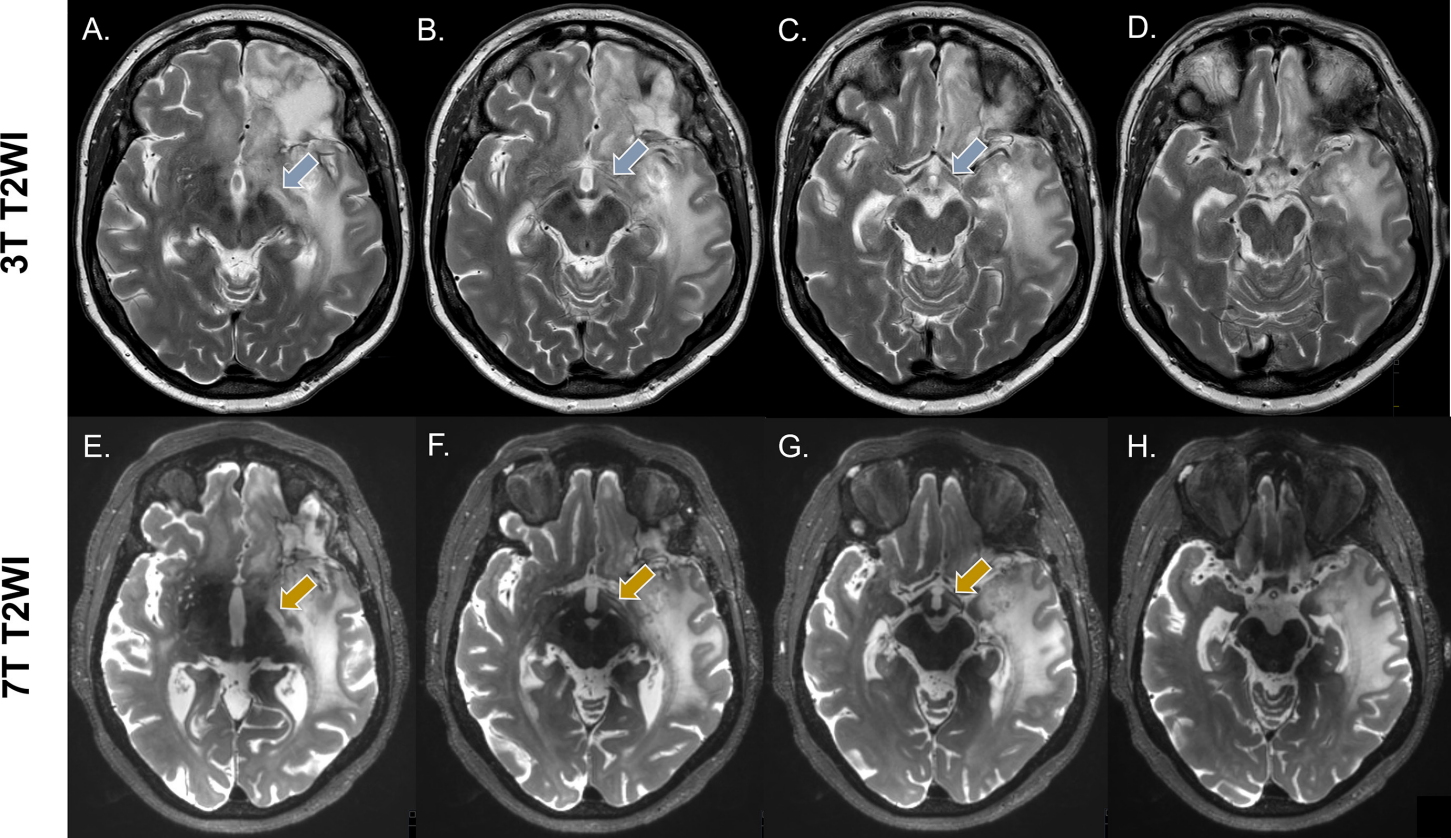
Visual assessment of tumor extension to the left optic tract using T_2_‐weighted images. An example of a patient with a glioblastoma, IDH‐wild‐type in whom there is a suspicion of involvement of the left optic tract in the tumor pathology. Whereas a T_2_ hyperintense area can be seen on the clinical scans, the connection between the left optic tract and the lesion can be better followed on the high‐resolution 7 T MRI scans.
